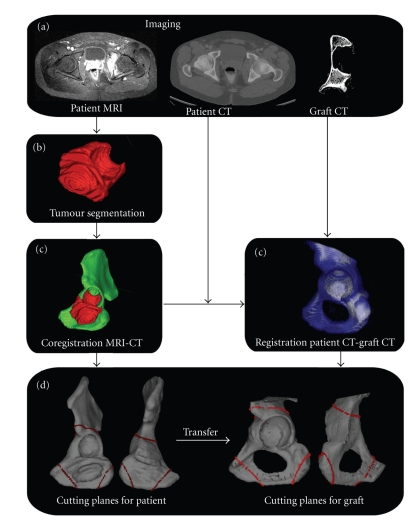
(a) Preoperative imaging workup of the patient consisted of MRI and CT of the pelvis. A CT of the allograft (iliac bone) was also obtained. (b) The tumor is segmented on the MRI slices. (c) The MRI and CT are coregistered. The CT of the patient and of the allograft are registered. (d) The target planes (red lines) are preoperatively defined by locating the planes with respect of a safe margin from the tumor. The planes coordinates are transferred to the allograft.
